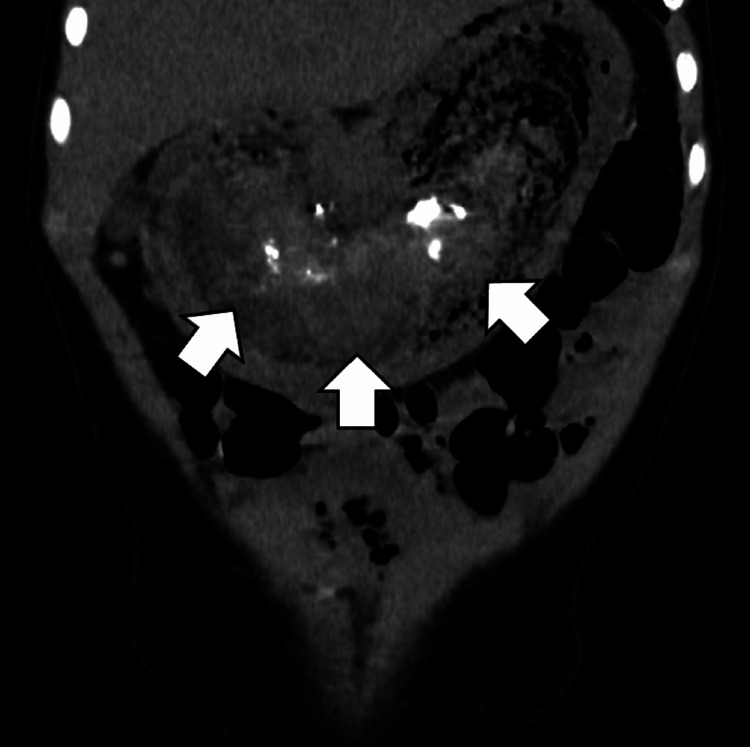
A preoperative coronal abdominal computed tomography scan showed a large bezoar (arrows).
